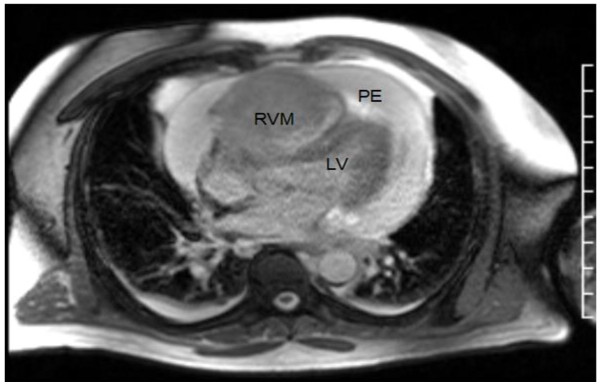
Cardiac MRI, T1 weighted bright blood imaging axial orientation.
